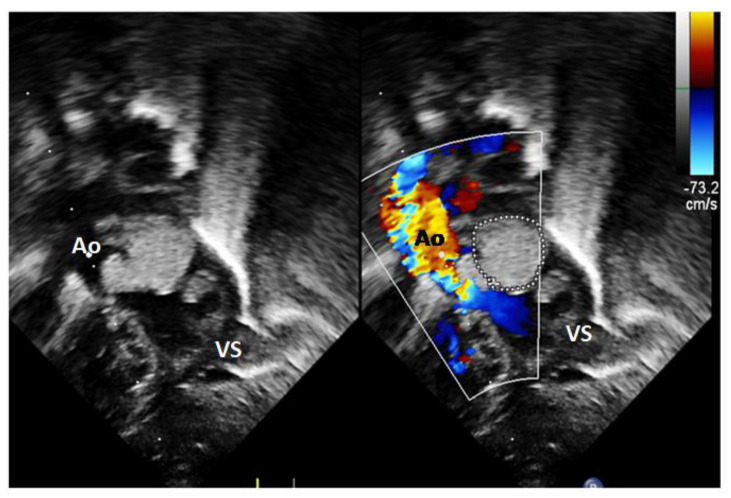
Echocardiography at birth: both echodense masses are seen, with a turbulent aortic flow. Two masses are evident in the left ventricle (LV), a bigger one in the inflow, surrounded by a circle, and a smaller one in the aortic outflow tract (x). VS—left ventricle, Ao—aorta.
